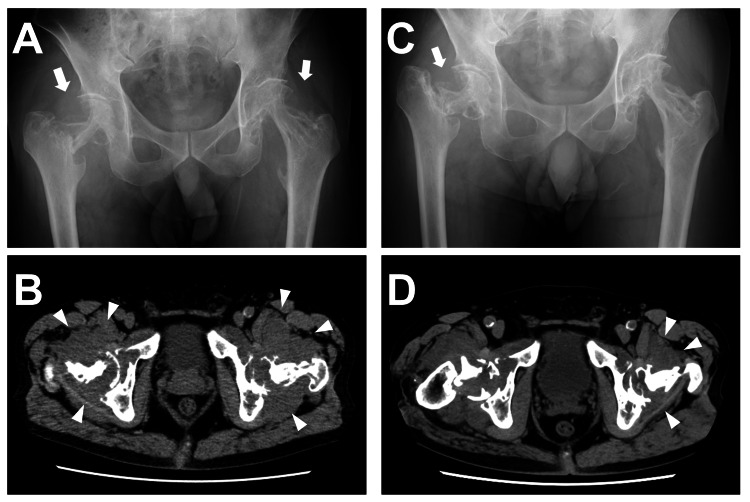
Changes in the hip joint lesions pre- and post-trametinib treatment The patient developed late-onset bone lesions in the femur 13 years following diagnosis (2021). A. A radiograph of the hip joint before trametinib therapy revealed osteolysis in the bilateral femoral necks (arrows). B. CT of the hip joint before trametinib administration showed bone destruction in both the femoral neck and the acetabulum. Additionally, soft-tissue density shadows were noted in the bilateral periarticular area (arrowheads). C. A radiograph of the hip joint one year after the trametinib treatment displayed shortening of the right femoral neck indicating fracture was evident (arrow). D. CT of the hip joint one year after starting trametinib showed a reduction of the periarticular lesions particularly on the left side (arrowheads).
